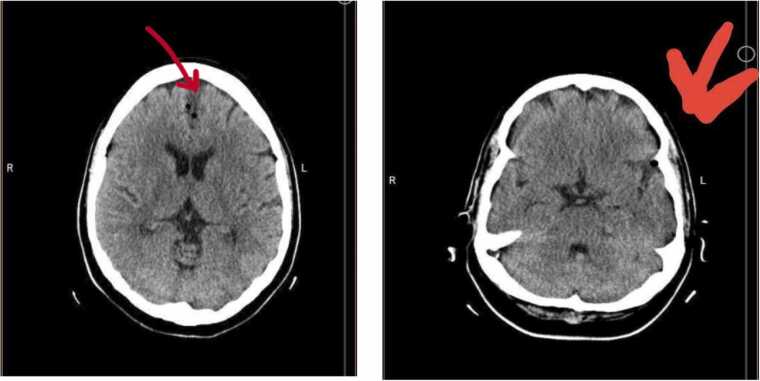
CT showing diffuse gas density in the brain parenchyma (red arrows).
